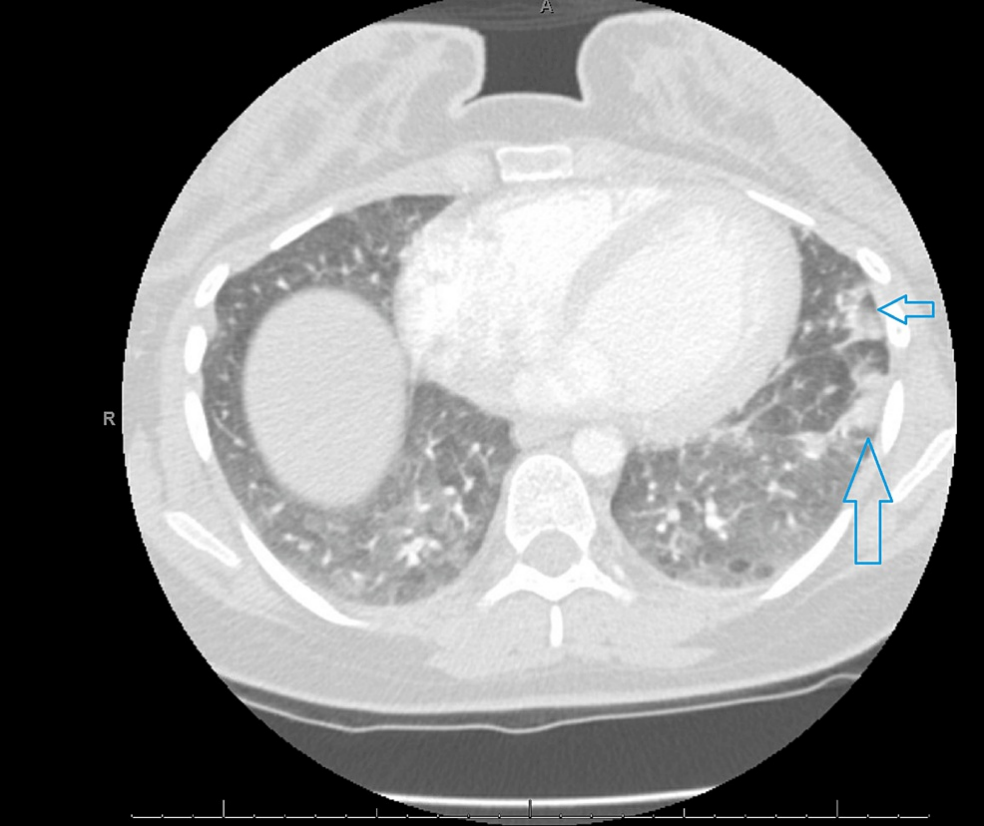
Patient's CT chest, lung window. Blue arrow: pneumonic patch
